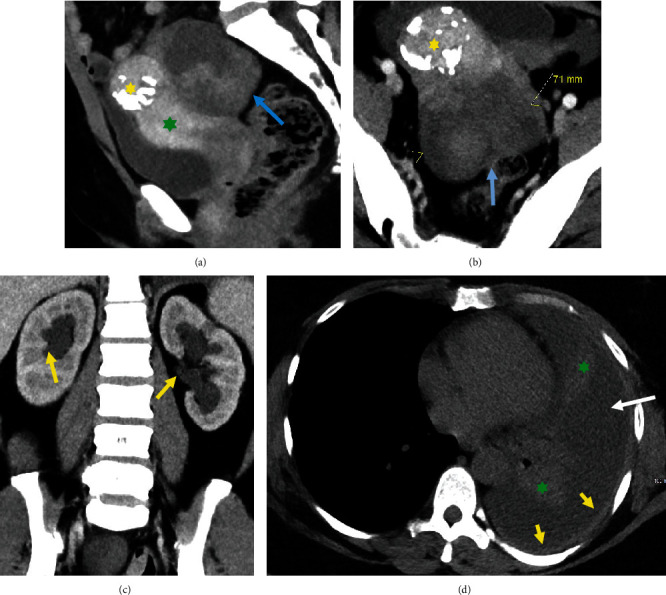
Sagittal (a) and axial (b) contrast-enhanced CT images of the pelvis demonstrate a large heterogeneous lobulated mass (blue arrow) posterior to the uterus (green asterisk). The uterus contains a coarsely calcified exophytic fibroid (yellow asterisk). (c) Bilateral mild-moderate hydroureteronephrosis with ureteral collapse at the level of the pelvic mass. (d) Axial unenhanced CT image of the chest demonstrates a large left pleural effusion (white arrow) with compressive atelectasis of the lingula and left lower lobe (green asterisk) as well as left posterior pleural thickening (yellow arrow).
